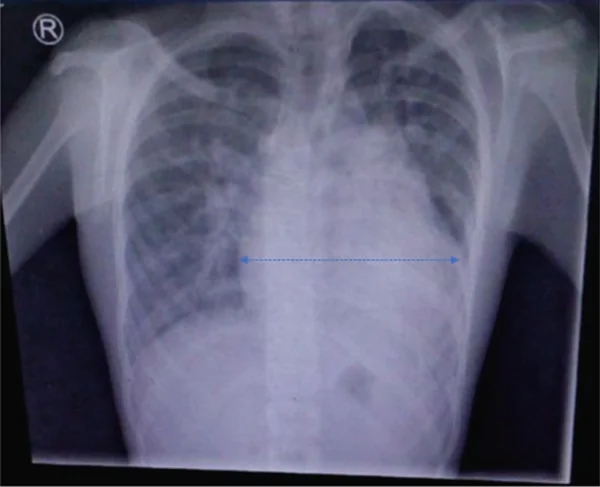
Posteroanterior chest radiograph showing cardiomegaly and Grade I pulmonary edema.
